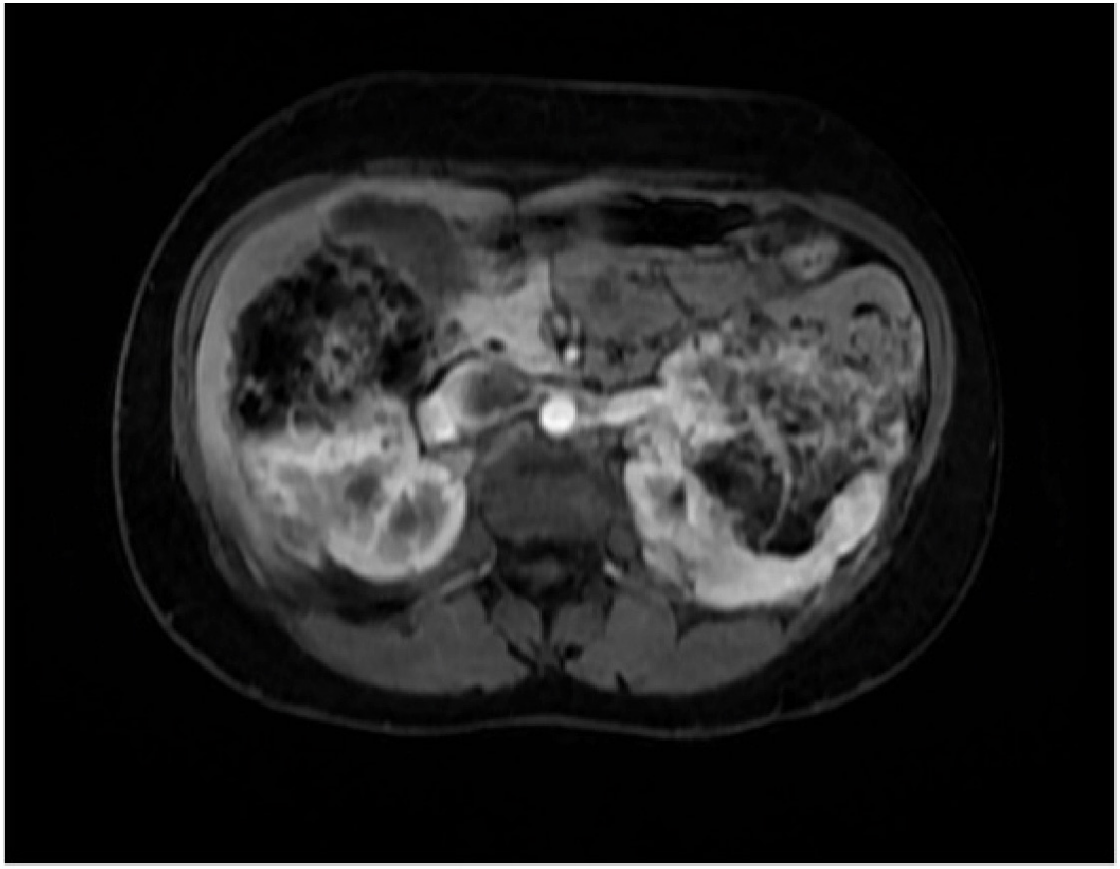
MRI showing large renal masses compatible with angiomyolipomas that replace practically the entire renal parenchyma in a patient with tuberous sclerosis complex.
